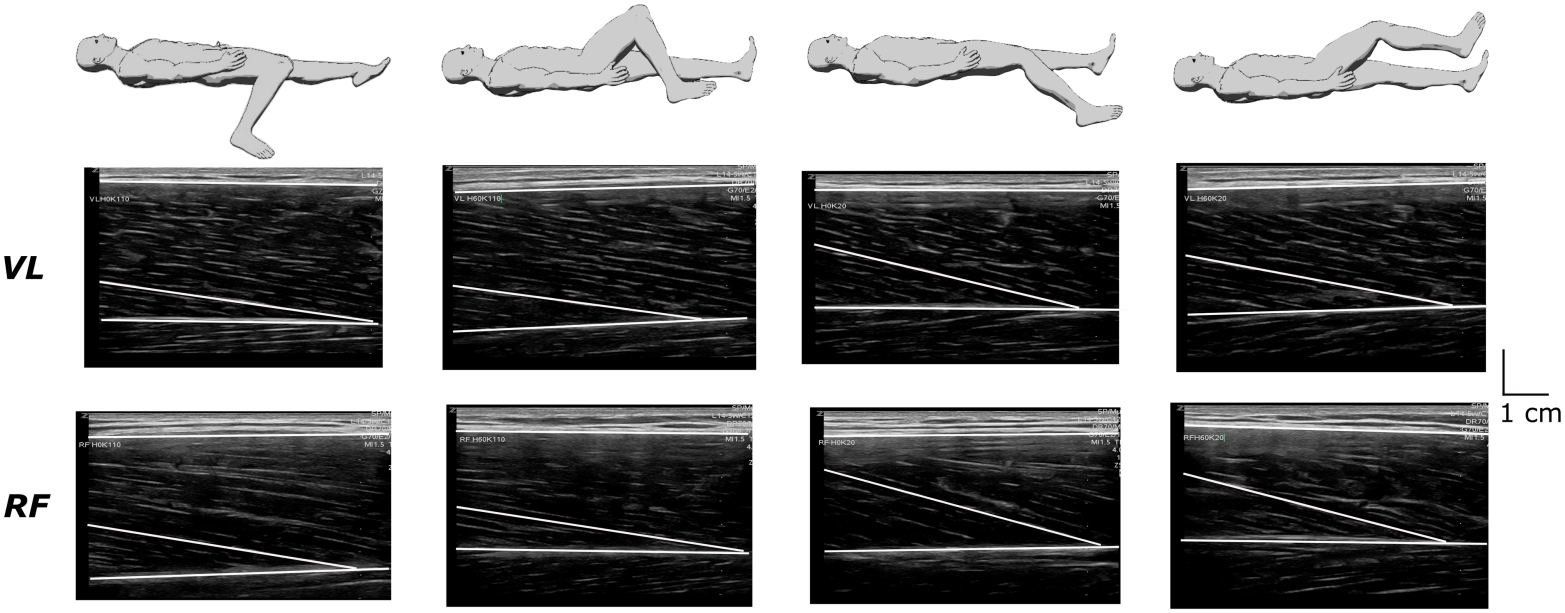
Recordings of ultrasound images from the vastus lateralis (VL) and the rectus femoris (RF) muscles under the different positions in a representative subject.
